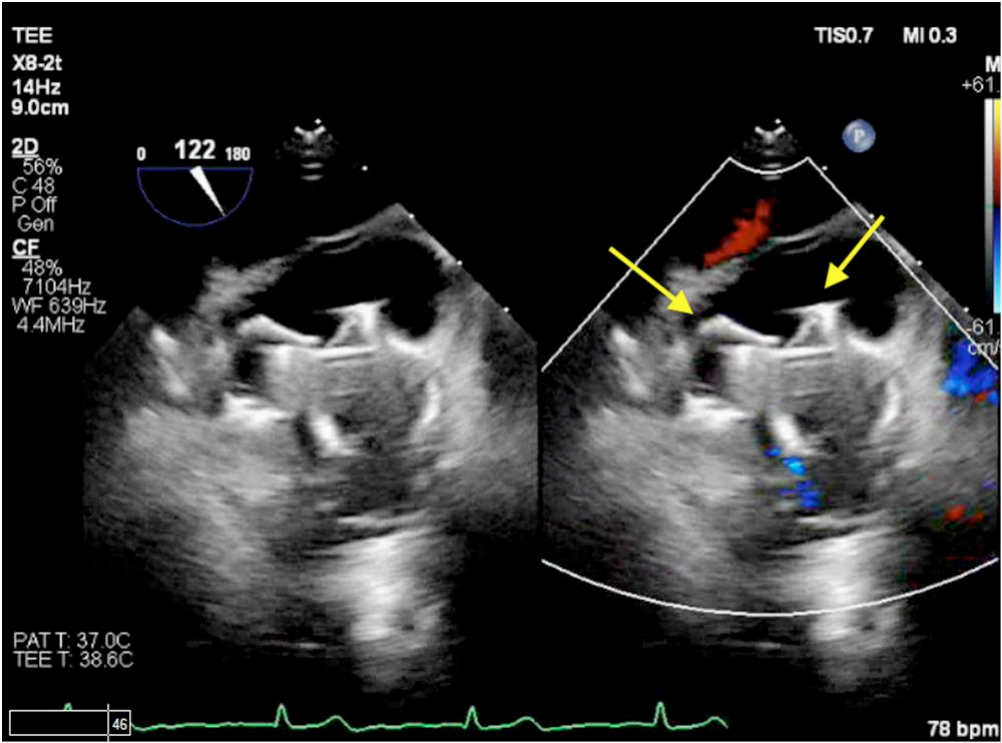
Transoesophageal echocardiogram with colour Doppler showing both WATCHMAN FLX devices (arrows) with no device thrombus and no peri-device leak.
